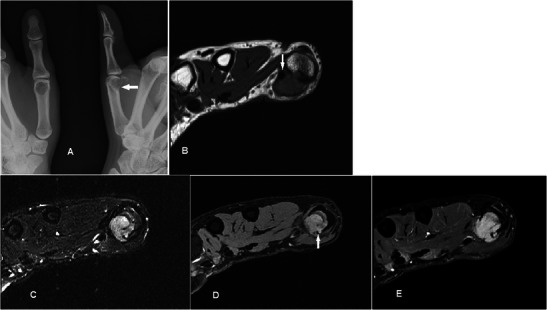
GCTTS in a 23-year-old female presenting with a 1-year history of right thumb swelling and mild pain. (a) Plain radiograph shows well-defined lucency at the head of the first metacarpal (arrow). (b) T1w sequence shows a lobulated subcutaneous lesion of intermediate signal encasing the flexor pollicis longus tendon (arrow). (c) The lesion shows heterogeneous high signal on T2w-FS sequence. There is pressure erosion on the underlying bone. (d) Foci of susceptibility are demonstrated on the GRE sequence (arrow). (e) There is avid and fairly homogeneous enhancement
